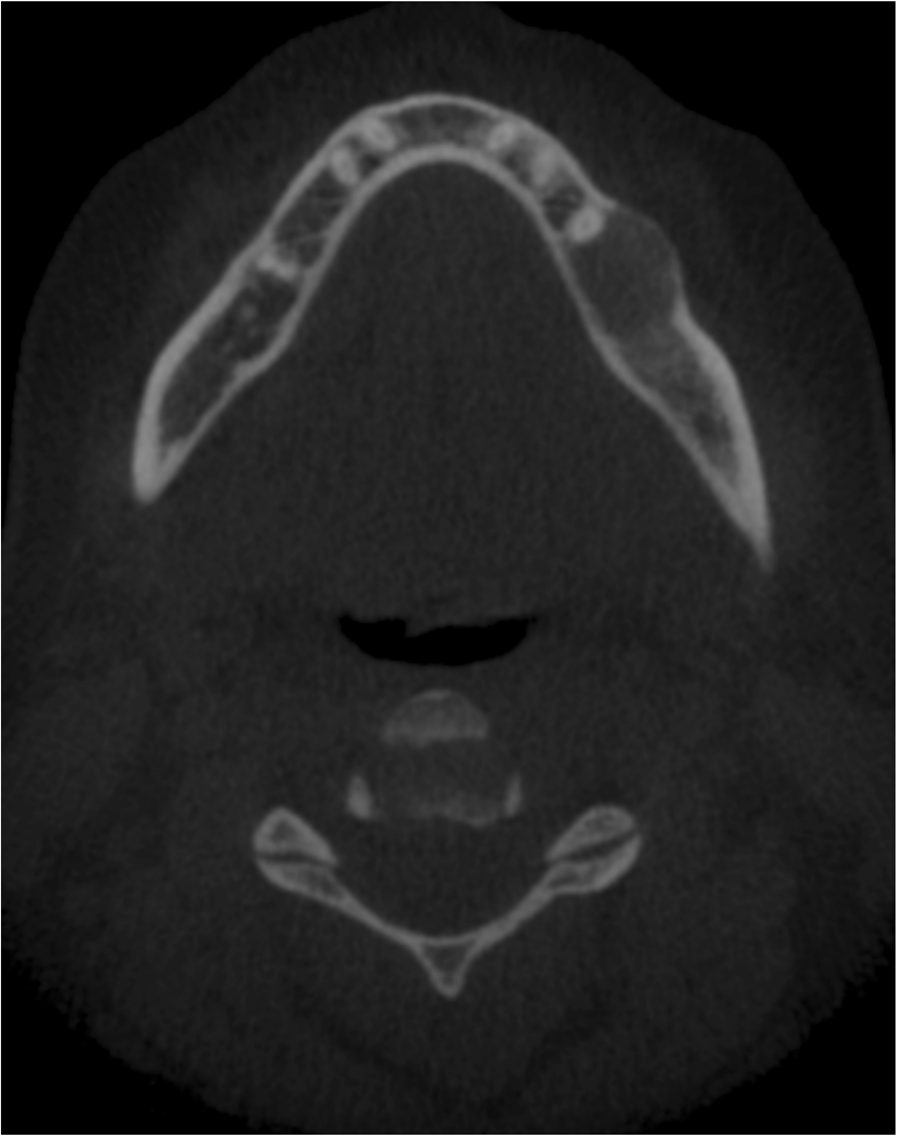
CT of the same patient showed a solitary expansile left mandibular low density lesion with associated cortical thinning
